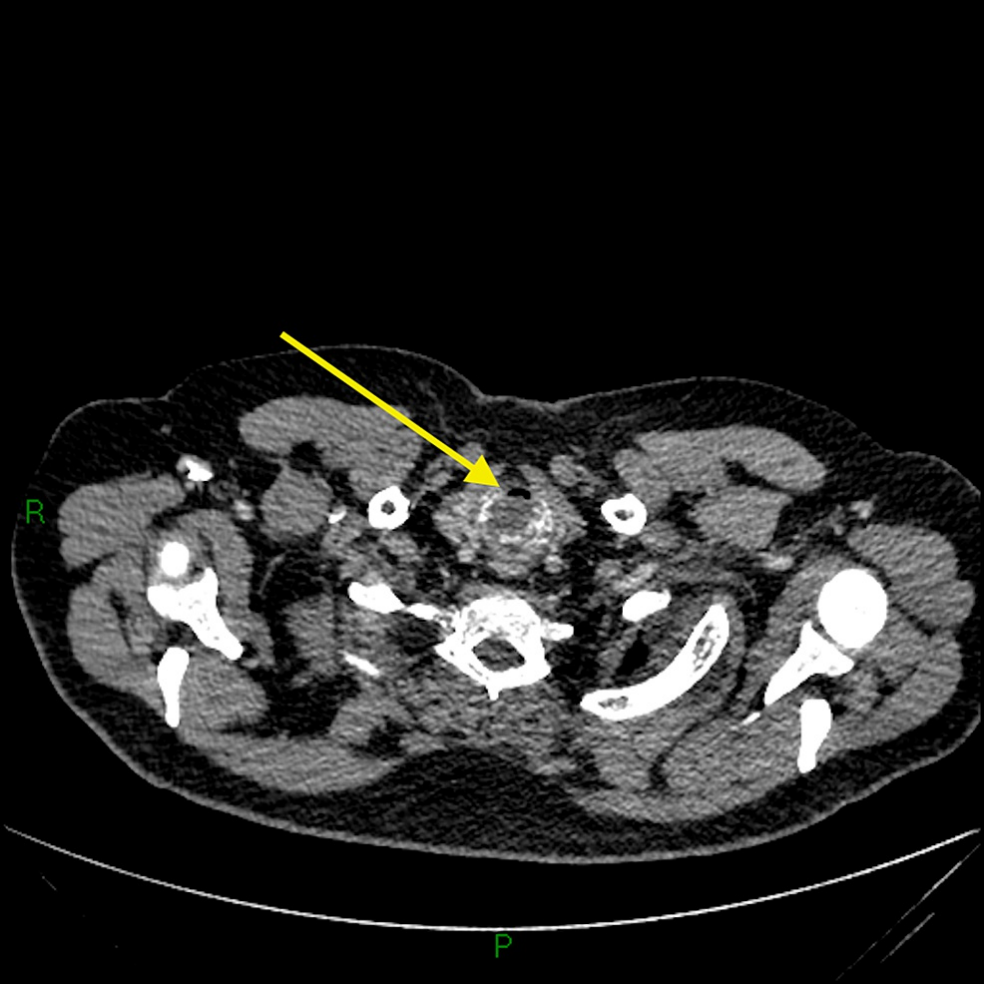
CT demonstrating the minimum diameter of the patient's airway. CT: computed tomography. This CT image demonstrates the minimum luminal airway dimension found which was 8 mm x 3 mm.
